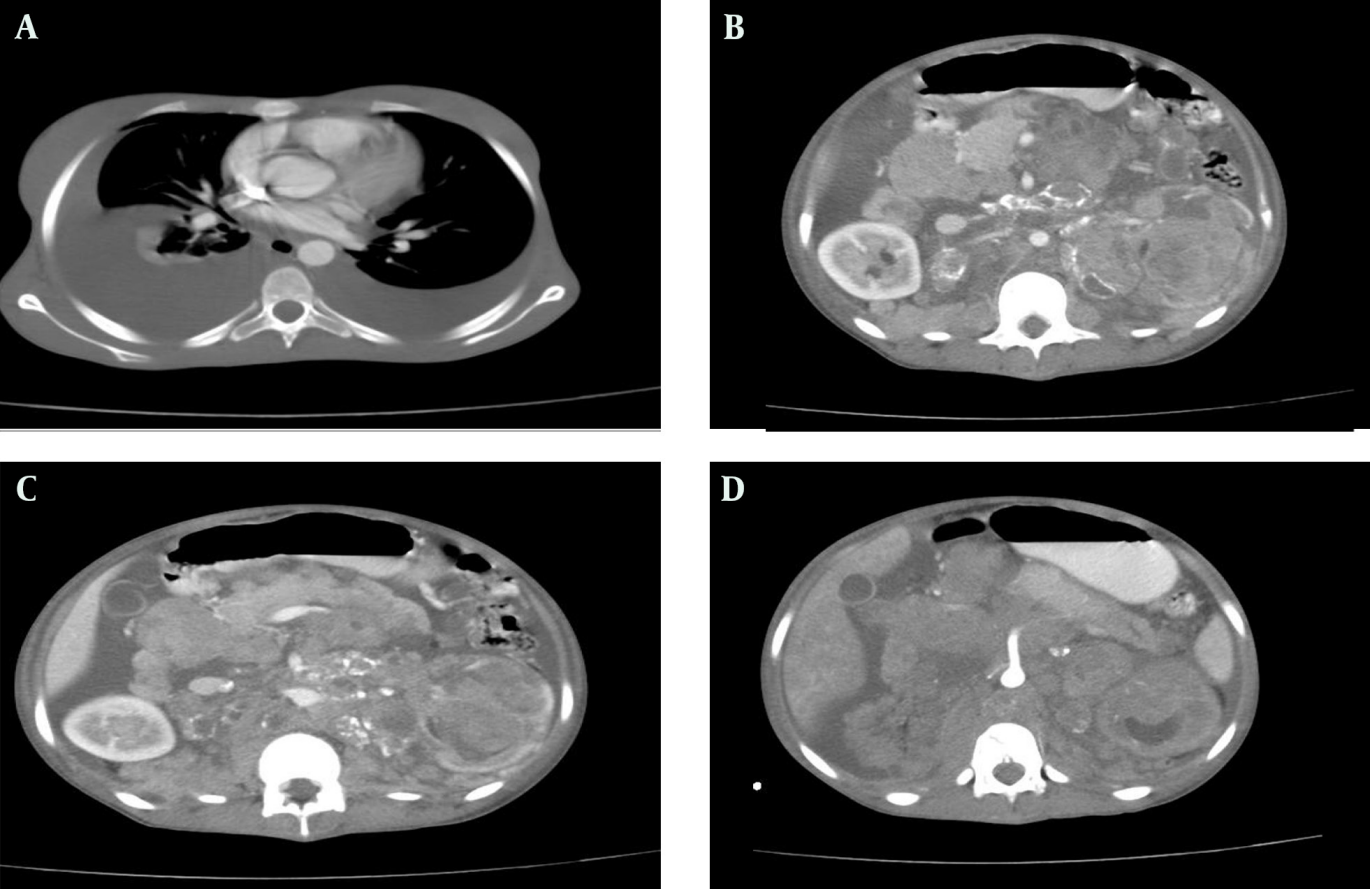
Thoracoabdominal CT scan of the patient with IV and oral contrast, A) Bilateral pleural effusion; B) Left kidney mass with heterogeneous enhancement; C) The pancreas is pushed anteriorly; D) Encasement of the superior mesenteric artery by mass lesion
